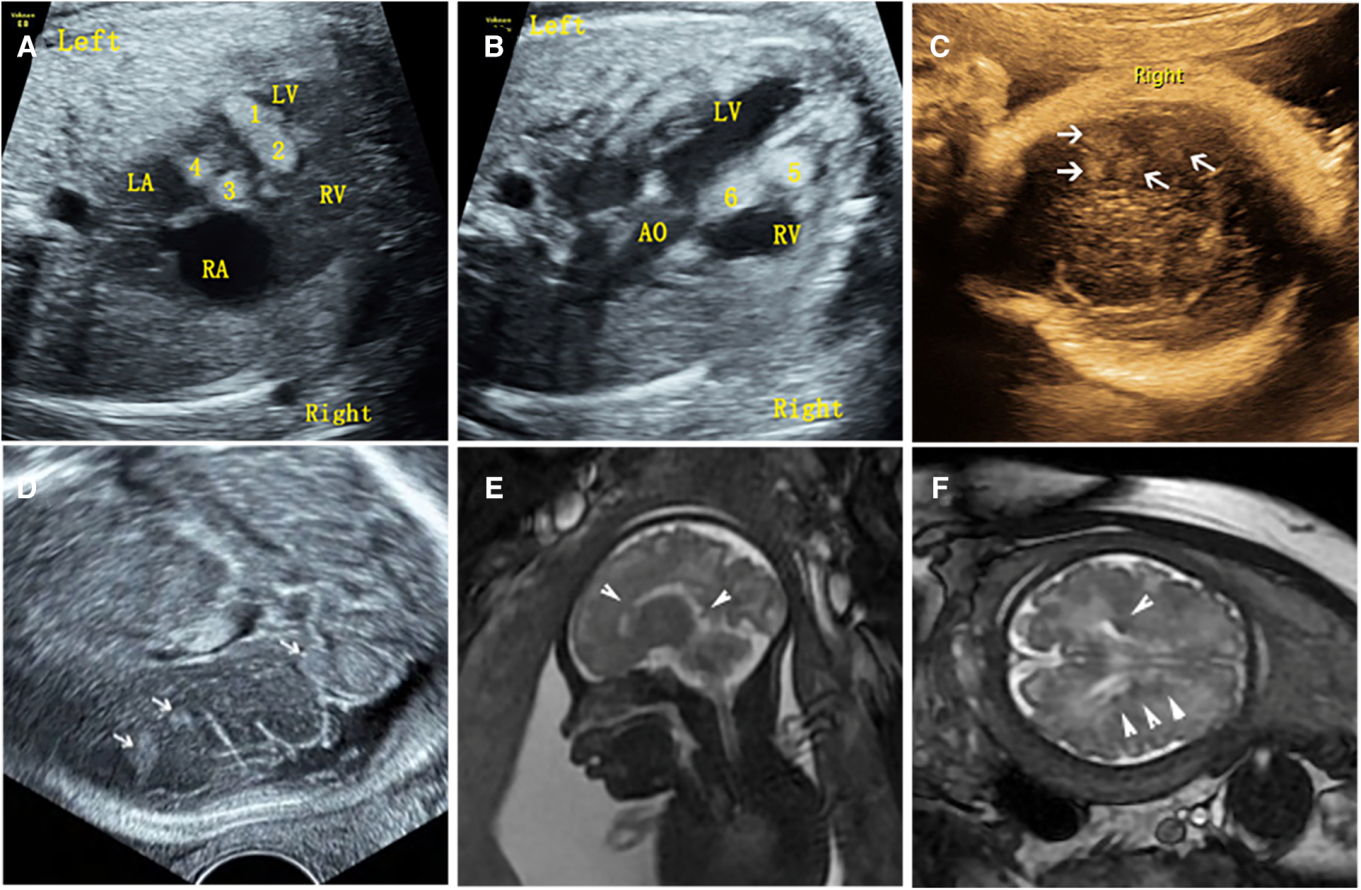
Ultrasound and MRI findings of multiple cardiac rhabdomyomas complicated with multiple cranial sclerotic nodules in the fetus (case NO. 51) at 31 weeks of gestation. (A) Multiple slightly hyperechoic nodules in the left ventricle of the fetal heart. (B) Multiple slightly hyperechoic nodules in the right ventricle of the fetal heart. (C) Multiple hyperechoic intracranial nodules of the fetus detected by transvaginal sonography. (D) Multiple hyperechoic intracranial nodules of the fetus detected by transabdominal sonography. (E) MRI sagittal T2-weighted image with multiple subependymal nodules. (F) MRI axial T2-weighted image with multiple subependymal nodules.
